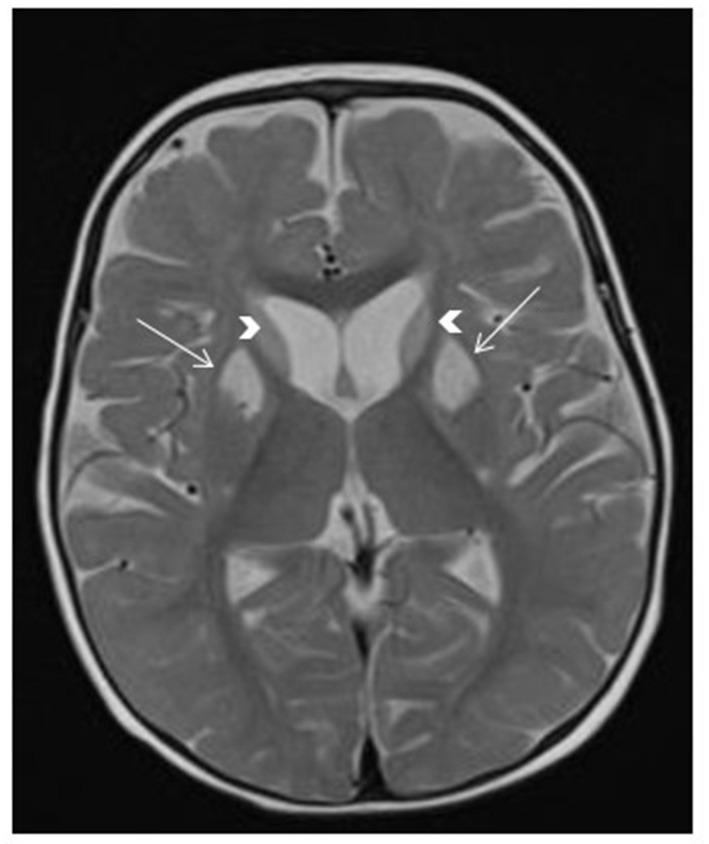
SERAC1*-related 3-methylglutaconic aciduria with deafness, encephalopathy and Leigh-like syndrome (MEGDEL). Brain MRI shows T2 hyperintensity in the bilateral caudate nucleus (arrowheads) and bilateral anterior putamen (arrows), associated with mildly enlarged anterior subarachnoid spaces.
